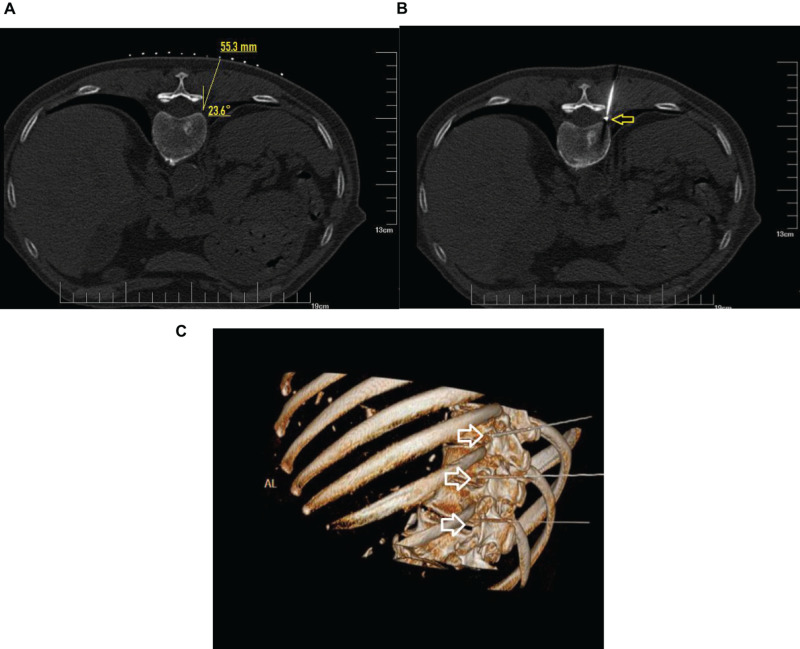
CT guidance: CT plain scan and 3D reconstruction. (A) Under CT guidance, a safe route was chosen to avoid injury to the vessel, pleura, and lung. The puncture point and puncture angle were clearly defined by computer. (B) CT scan showed that the radio frequency needle was located at the spinal nerve root on the left side and adjacent to the pleura and lung as shown by arrow. (C) The puncture needles of the three segments of the thoracic vertebrae were located at the intervertebral foramen as shown by the arrow.
